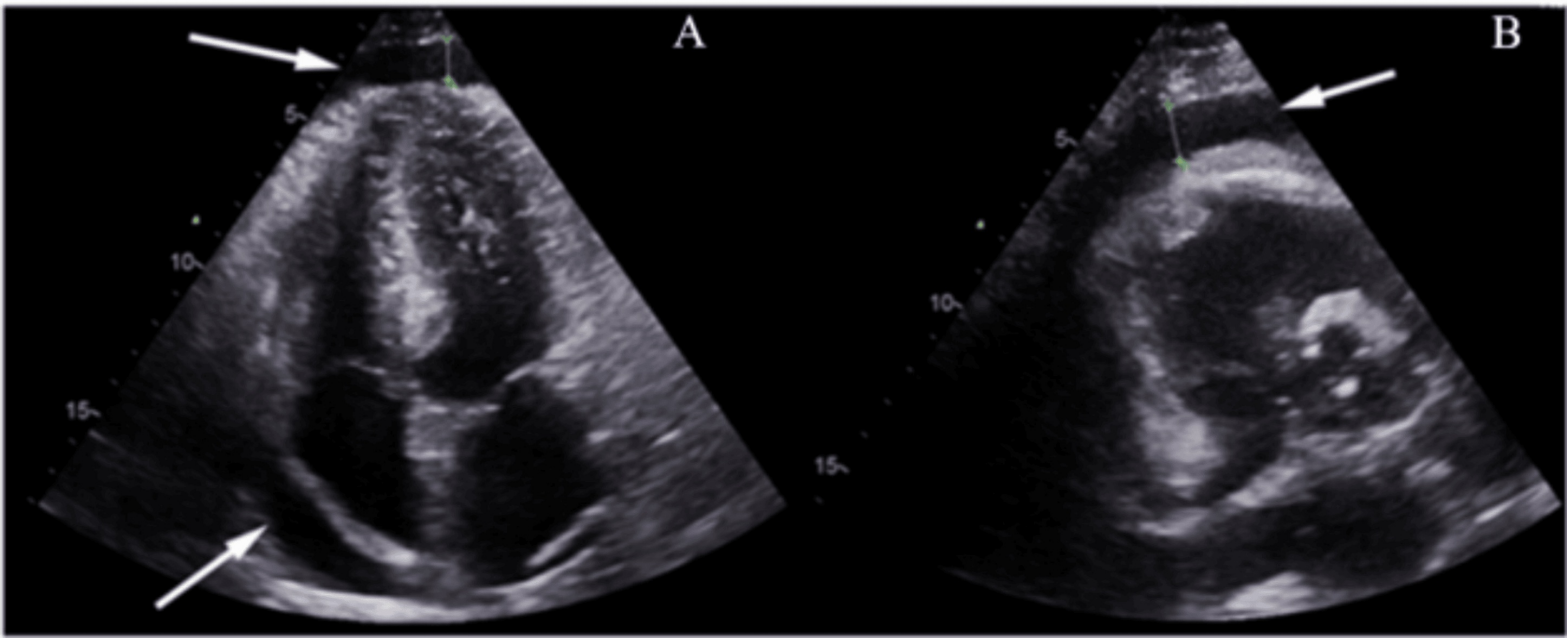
Transthoracic echocardiogram (TTE) A and B show a TTE demonstrating pericardial effusion. A: Apical four-chamber view showing a large anechoic space surrounding the heart, consistent with a circumferential pericardial effusion (white arrows). B: Parasternal long-axis view also revealing a significant pericardial effusion (white arrows), most prominent posteriorly.
